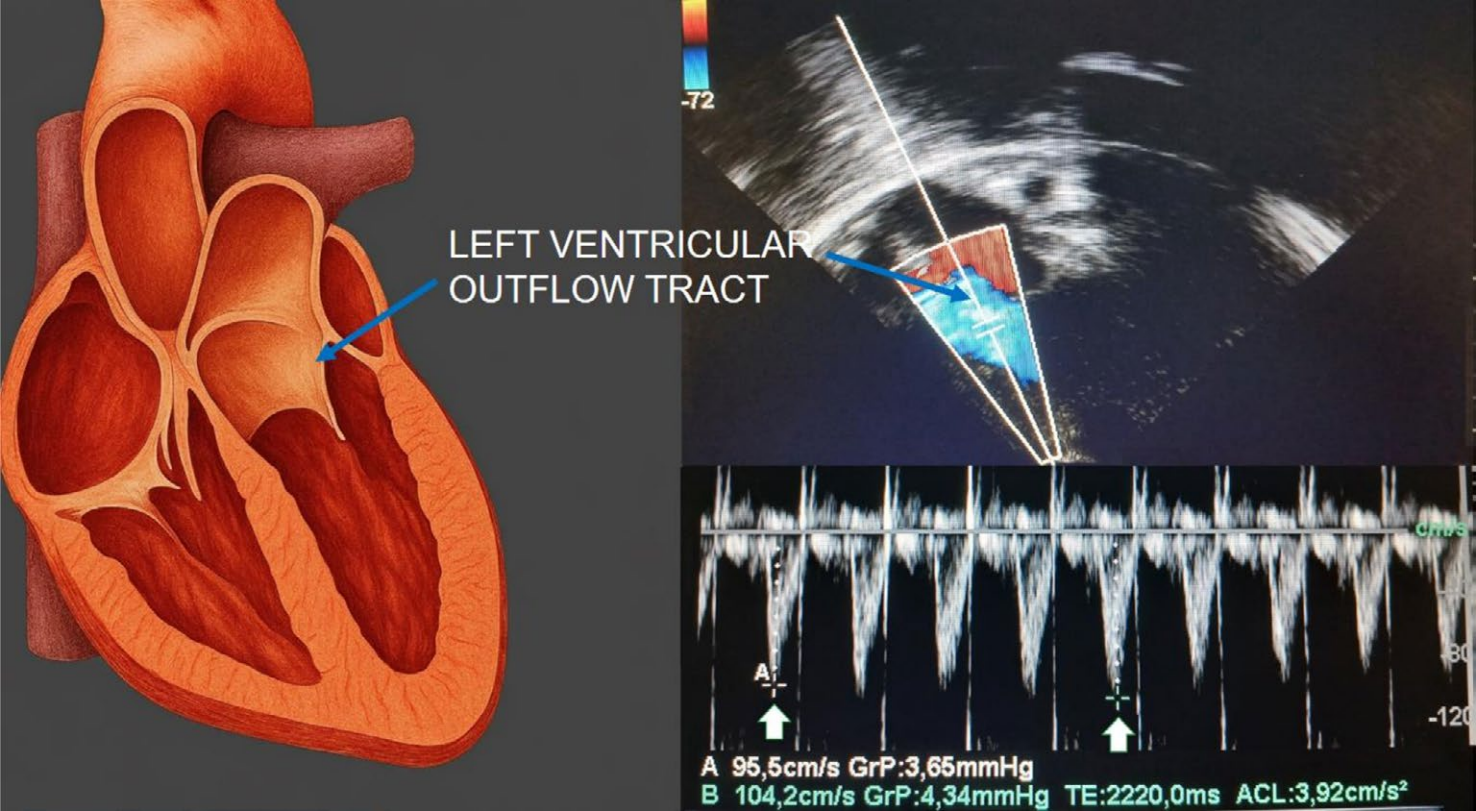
Method of measuring aortic flow velocities, with pulsed Doppler placed in the left ventricular outflow tract.
